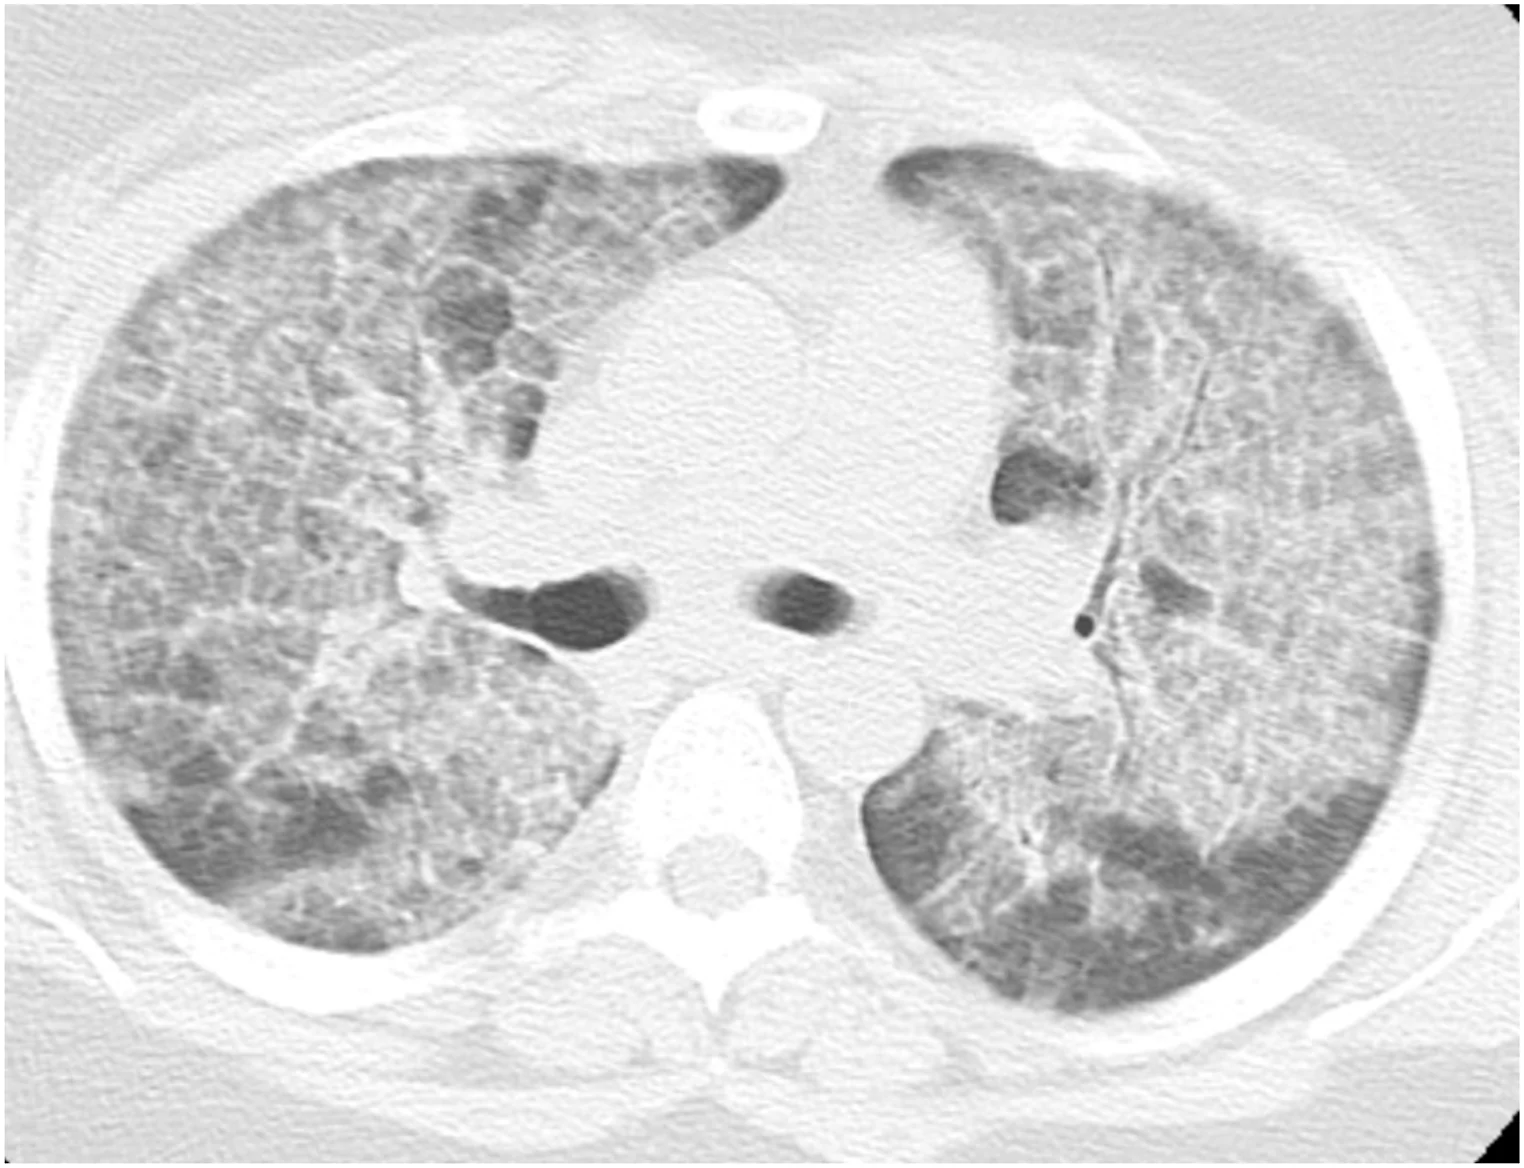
CT Chest without Contrast on subsequent admission showing increased multifocal opacities.
